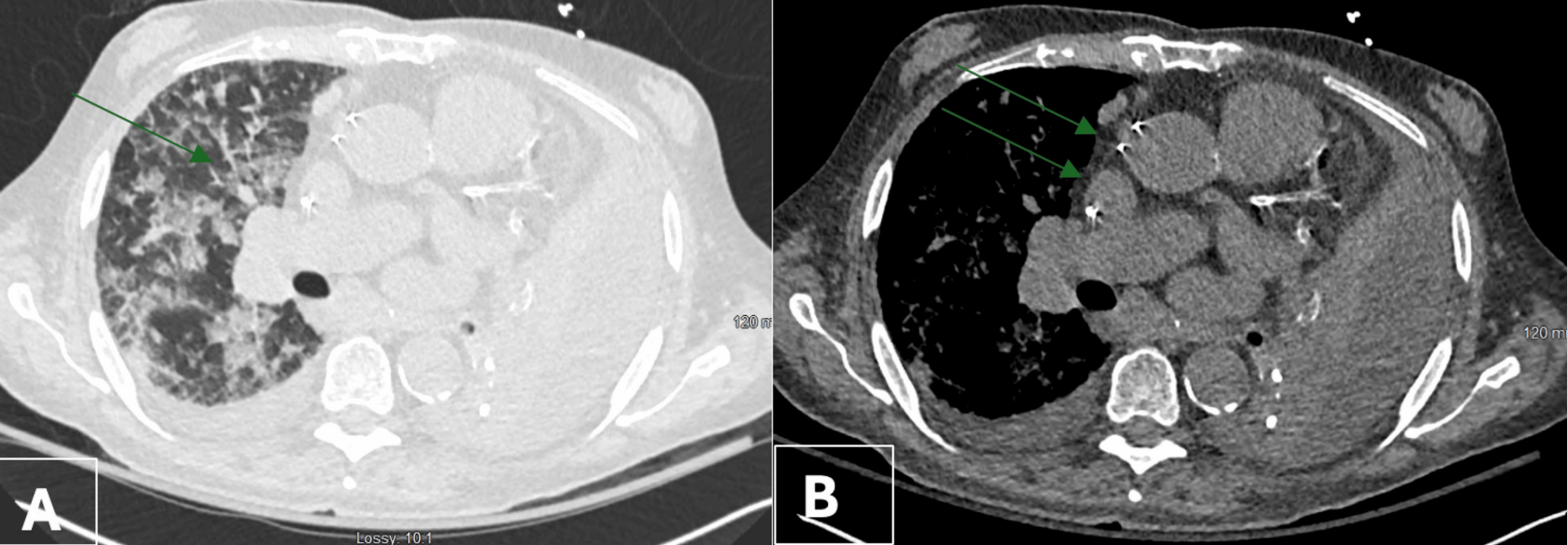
Computed tomography findings in Case 4. Axial contrast-enhanced chest CT images demonstrate left lower lobe collapse prior to intervention, consistent with post-obstructive pneumonia (A, lung window, arrow). Post-intervention imaging (B, mediastinal window) shows restoration of airway patency and re-expansion of the left lower lobe following bronchoscopic removal of an obstructing broncholith (arrows).
